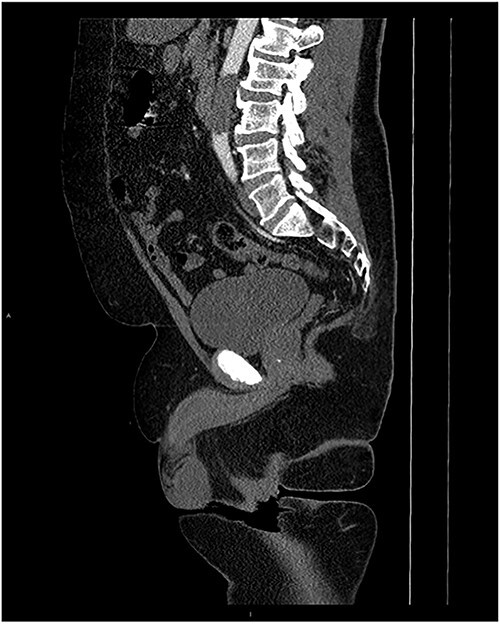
MRI Sagittal with contrast with occlusion of the abdominal aorta.
